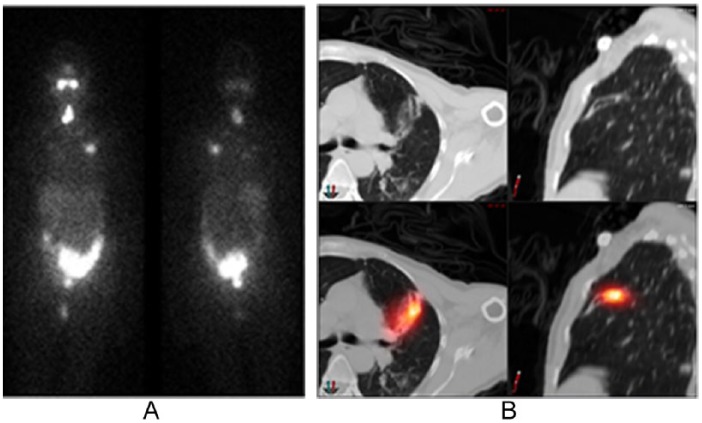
Cryptic uptake in the chest, corresponding to bronchiectasis on SPECT/CT. A 56-year-old woman with a history of PTC treated with surgery and then 100 mCi of I-131. Posttherapy WBS (4A) demonstrates focal activity in the lungs. On SPECT/CT (4B), this area was diagnosed as bronchiectasis. Abbreviations: WBS, whole body scan; SPECT/CT, single-photon emission computed tomography.
